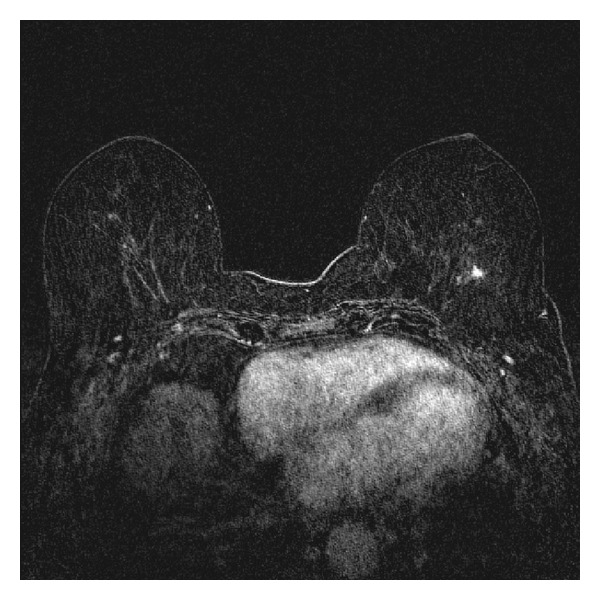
Subtraction MRI demonstrates a 0.9 cm enhancing mass with spiculated borders within the posterior left breast with no involvement of the left chest wall. This posterior mass was not appreciated on mammography. Under MRI guidance, this mass was biopsy proven to represent invasive pleomorphic lobular carcinoma.
